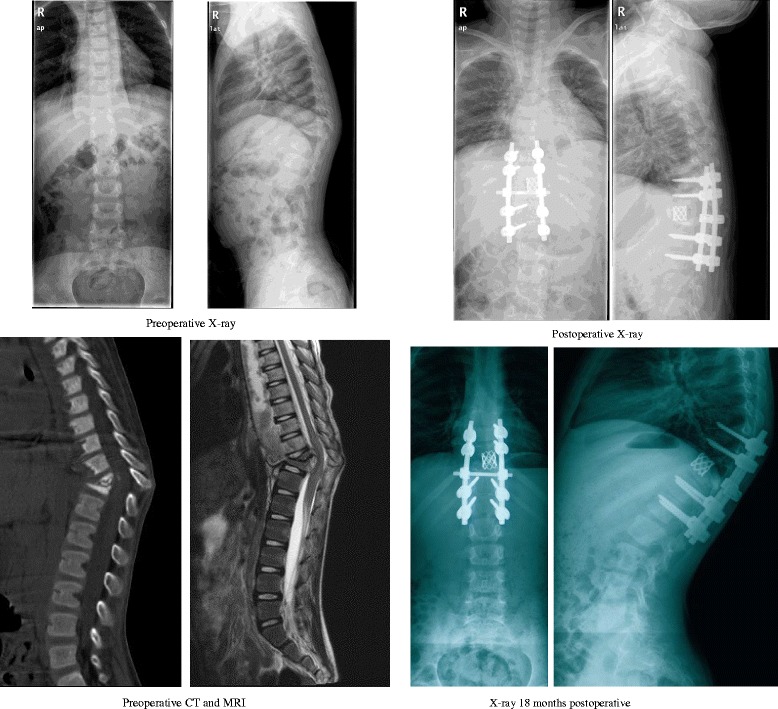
Female, 8 years, T9, T10, and T11 TB with paravertebral cold abscess formation. Preoperative X-ray AP and lateral view, kyphotic Cobb angle of 46°; preoperative two-dimensional sagittal CT, T10, T11 vertebral severe destruction; preoperative MR, spinal cord was compressed obviously in T10 and T11 levels with paravertebral cold abscess formation; postoperative X-ray AP and lateral view, kyphotic Cobb angle of 10°; X-ray AP view 18 months postoperative: T8 vertebral pedicle screw cutting, proximal adjacent kyphosis, Cobb angle 46°
